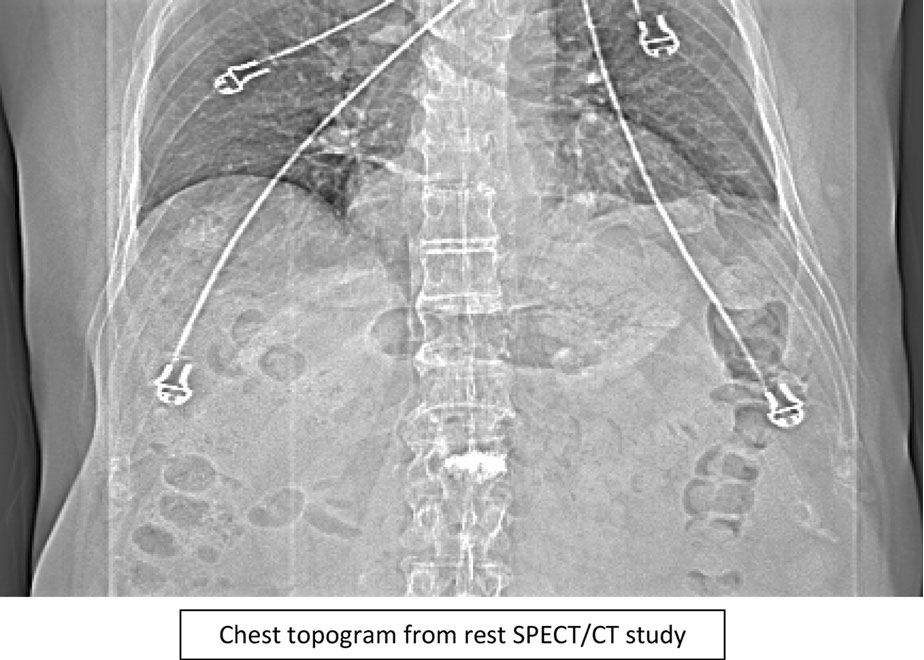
Chest topogram from rest SPECT/CT study
